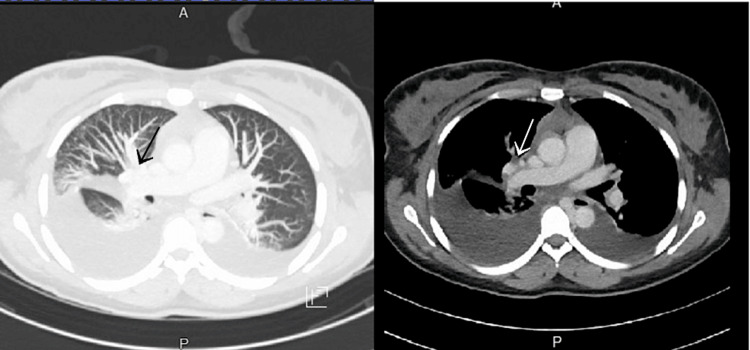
CT scan of the chest with and without contrast demonstrating a mass in the anterior superior mediastinal region which is encasing around the brachiocephalic veins and superior vena cava (SVC), accompanied by bilateral pleural effusion which is more pronounced on the right side.
